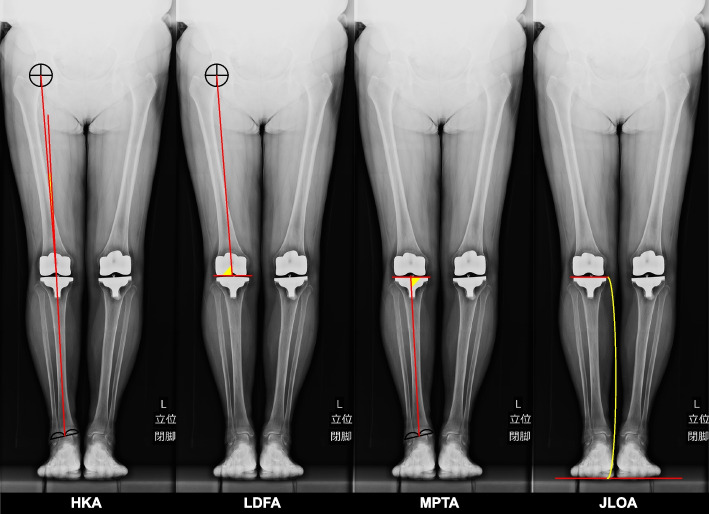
Measurements on closed-leg standing long leg radiographs. Abbreviations: HKA: hip-knee-ankle angle; JLOA: joint line orientation angle; LDFA: lateral distal femoral angle; MPTA: medial proximal tibial angle
